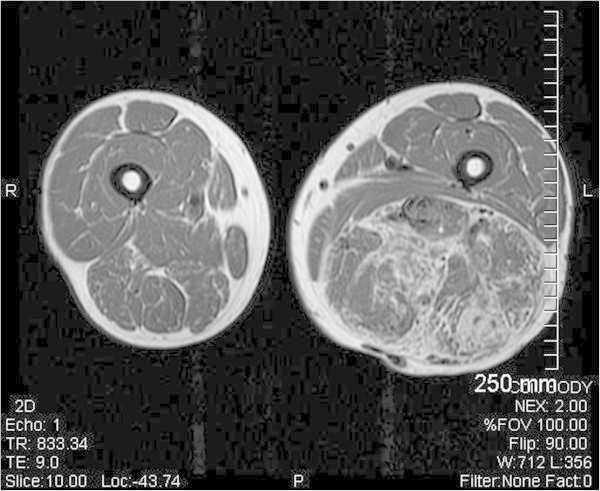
T1-weighted axial MR image with fat saturation shows an encapsulated soft tissue mass with in the left biceps femoris muscle.
